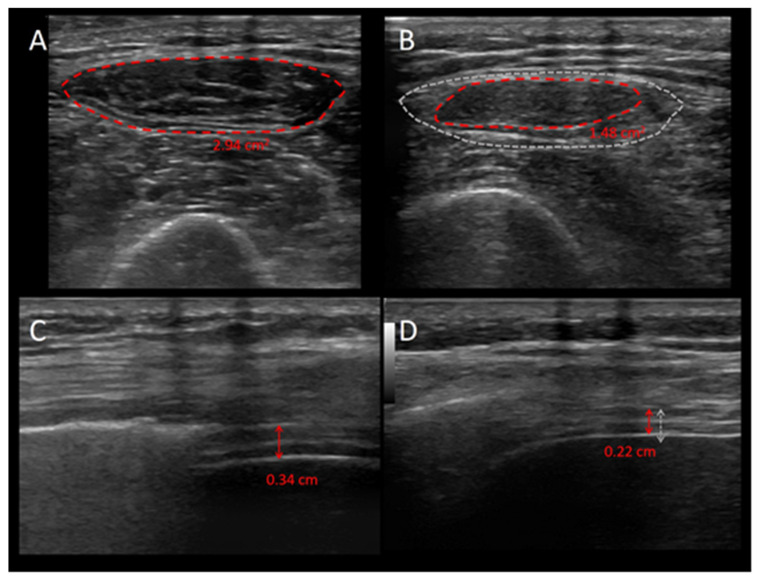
Ultrasonographic images of the quadriceps muscle and the diaphragm during the first week of ICU stay of a critically ill patient. The figure shows the images of the quadriceps muscle (upper panels (A,B)) and the diaphragm (lower panels (C,D)) of a critically ill patient during the first (left panels) and seventh day of ICU stay (right panels) and highlights the loss in muscle mass. Upper panels: The red, shaded line represents the cross-sectional area of the rectus femoris muscle; the grey, shaded area in panel (B) is the cross-sectional area of the first ICU day, and highlights the reduction in mass of the muscle. Lower panels: the red arrows show the diaphragm end-expiratory thickness; the grey, shaded line in panel (D) is the end-expiratory diaphragm thickness of the first ICU day.
